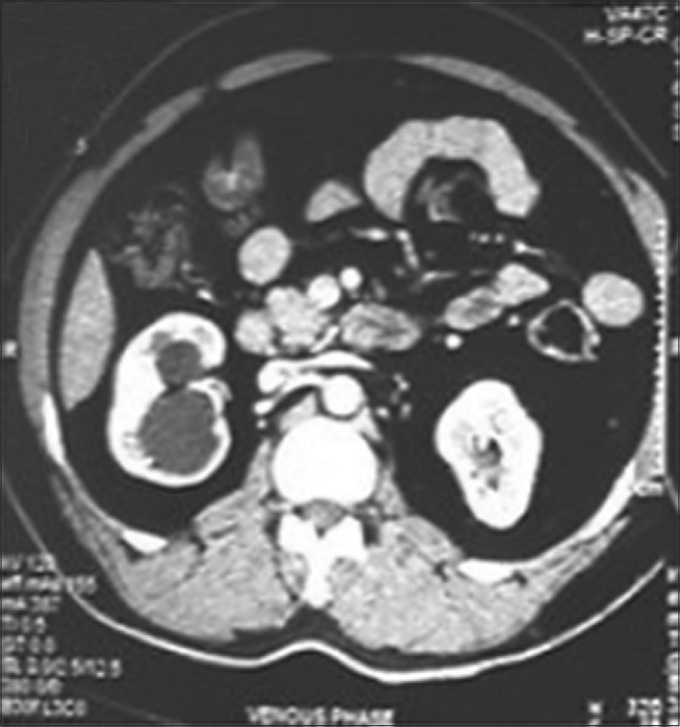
Preoperative CT scan showing normal renal parenchyma
